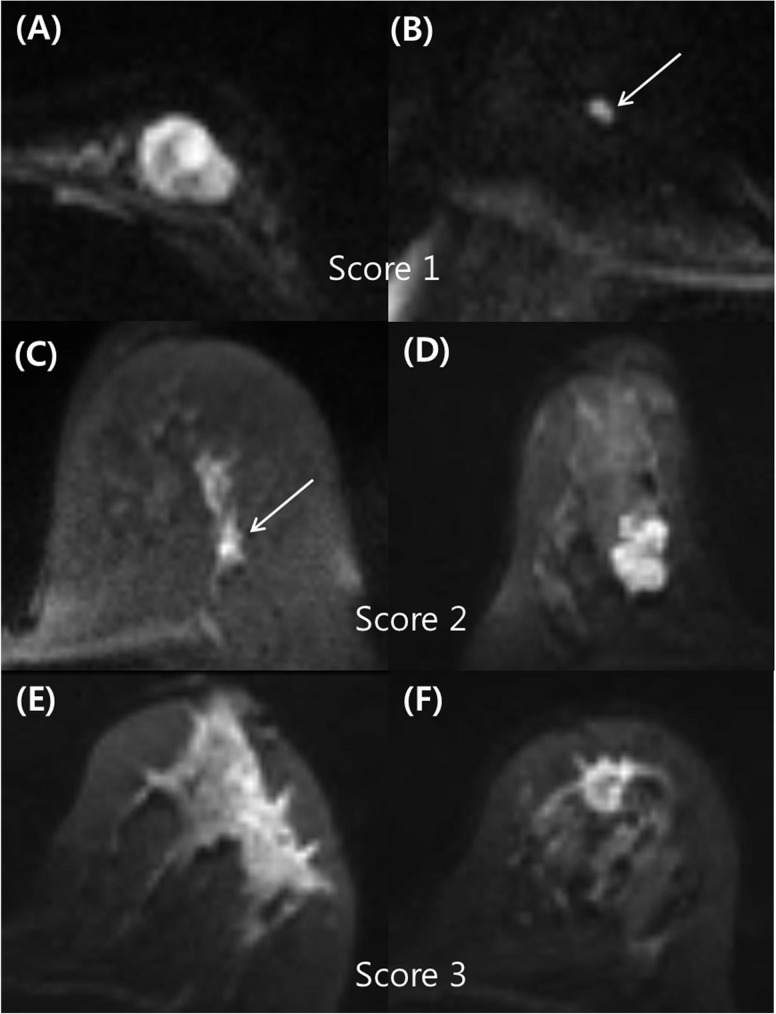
Examples of typical cases for each type of score. (A) A 43-year-old woman with a mucinous carcinoma in the left breast. The rs-EPI DWI demonstrated an oval circumscribed mass with a heterogeneous internal pattern in the left breast, which was scored as 1 (probably benign finding) during qualitative analysis. (B) An 80-year-old woman with invasive ductal carcinoma in the right breast. The rs-EPI DWI demonstrated an oval circumscribed mass with a homogeneous internal pattern in the right breast, which was scored a 1 (probably benign finding) on qualitative analysis. (C) A 61-year-old woman with ductal carcinoma in situ of the left breast. During qualitative analysis of rs-EPI DWI, there was a focal heterogeneous non-mass lesion, which was scored a 2 (indeterminate finding). (D) A 44-year-old woman with invasive ductal carcinoma in the right breast. The rs-EPI DWI demonstrated an irregular mass with an irregular margin and a homogeneous internal pattern, which was scored a 2 (indeterminate finding). (E) A 59-year-old woman with invasive ductal carcinoma of the left breast. During qualitative analysis of rs-EPI DWI, there was a segmental heterogenous non-mass that was scored a 3 (probably malignant finding). (F) A 51-year-old woman with invasive ductal carcinoma of the left breast. During qualitative analysis of rs-EPI DWI, there was an irregular spiculated mass with rim sign, which was scored a 3 (probably malignant finding).
